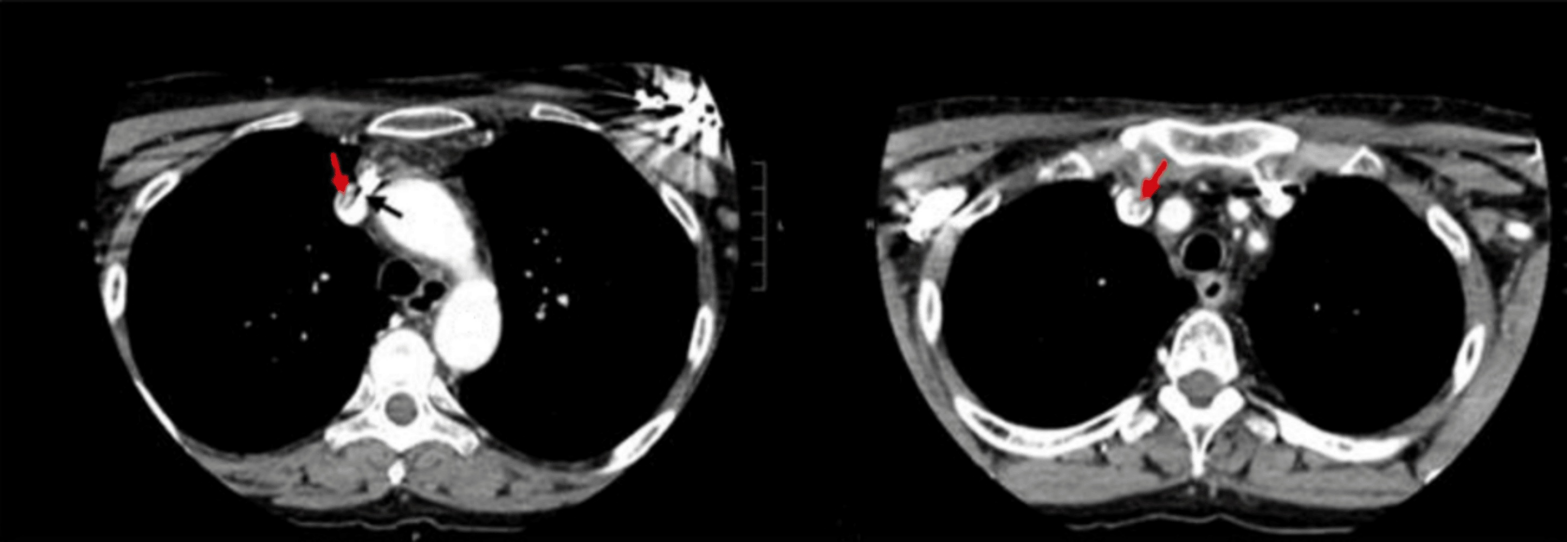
Axial CT neck with IV contrast, demonstrating stricture formation (red arrow) within the superior vena cava, with adjacent pacemaker lead (black arrow). CT: computed tomography.
